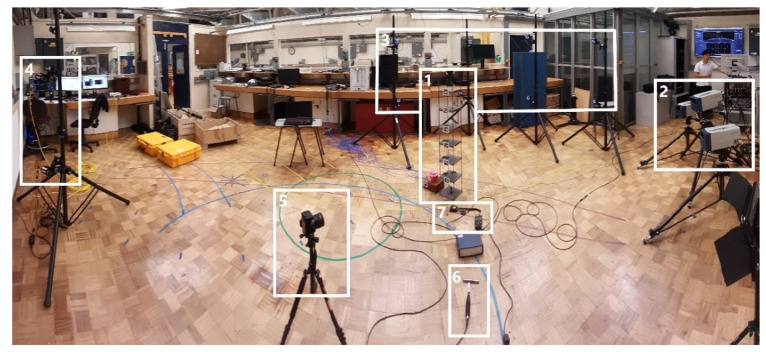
The layout of instrumentation systems in the Dynamics, Vibration, and Acoustics Laboratory at the University of Leicester: 1—Steel frame with accelerometers attached; 2—Polytec laser Doppler vibrometer; 3—OptiTrack; 4—Imetrum; 5—Consumer-grade camera (CGC); 6—instrumented hammer; 7—lux meter.
